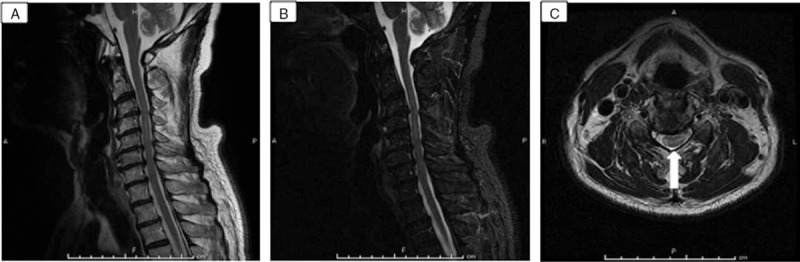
Magnetic resonance imaging (MRI) findings on hospital day 20. A, Sagittal T2-weighted (3516.67/87.65 [TR/TE]) and (B) short T1 inversion recovery (STIR; 4100/85.27 [TR/TE]) images. C, Hyperintense signal persists at C4/5 on axial T2-weighted (3500/109.20 [TR/TE]) image (arrow).
